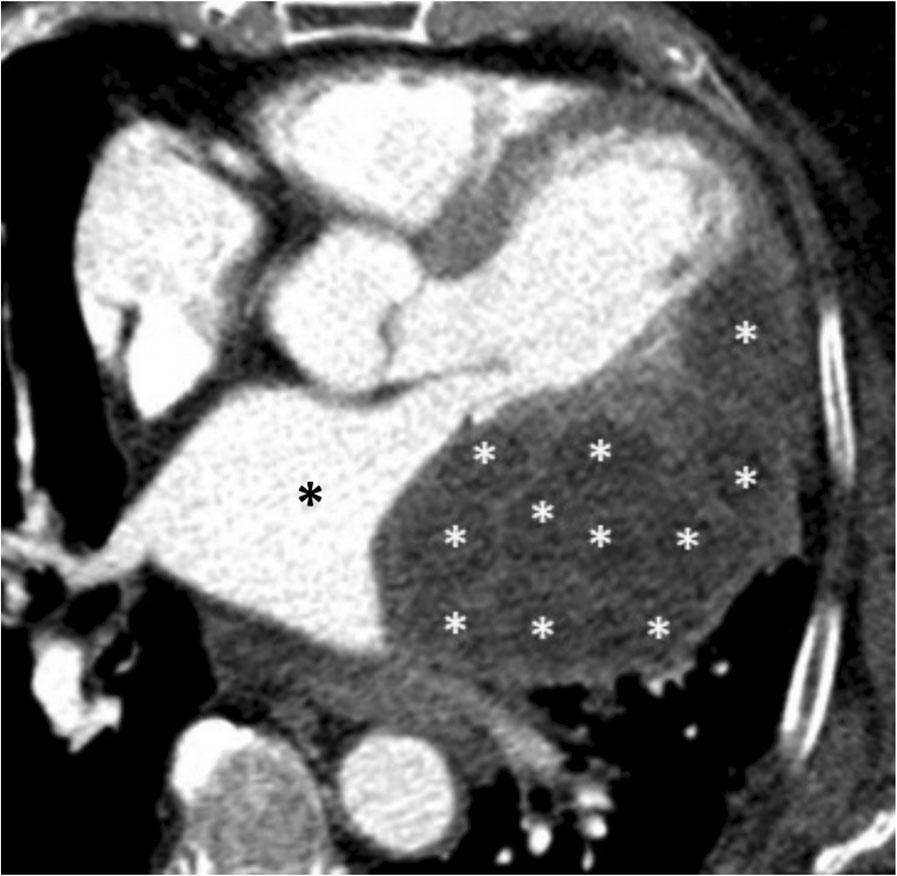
A 86-year-old man with hydatid disease. Axial contrast-enhanced CT image reveals type 3 hydatid cyst containing multiple daughter cysts (white asterisks). Significantly compressed left atrium (black asterisk) due to hydatid cyst abutting the pericardium
